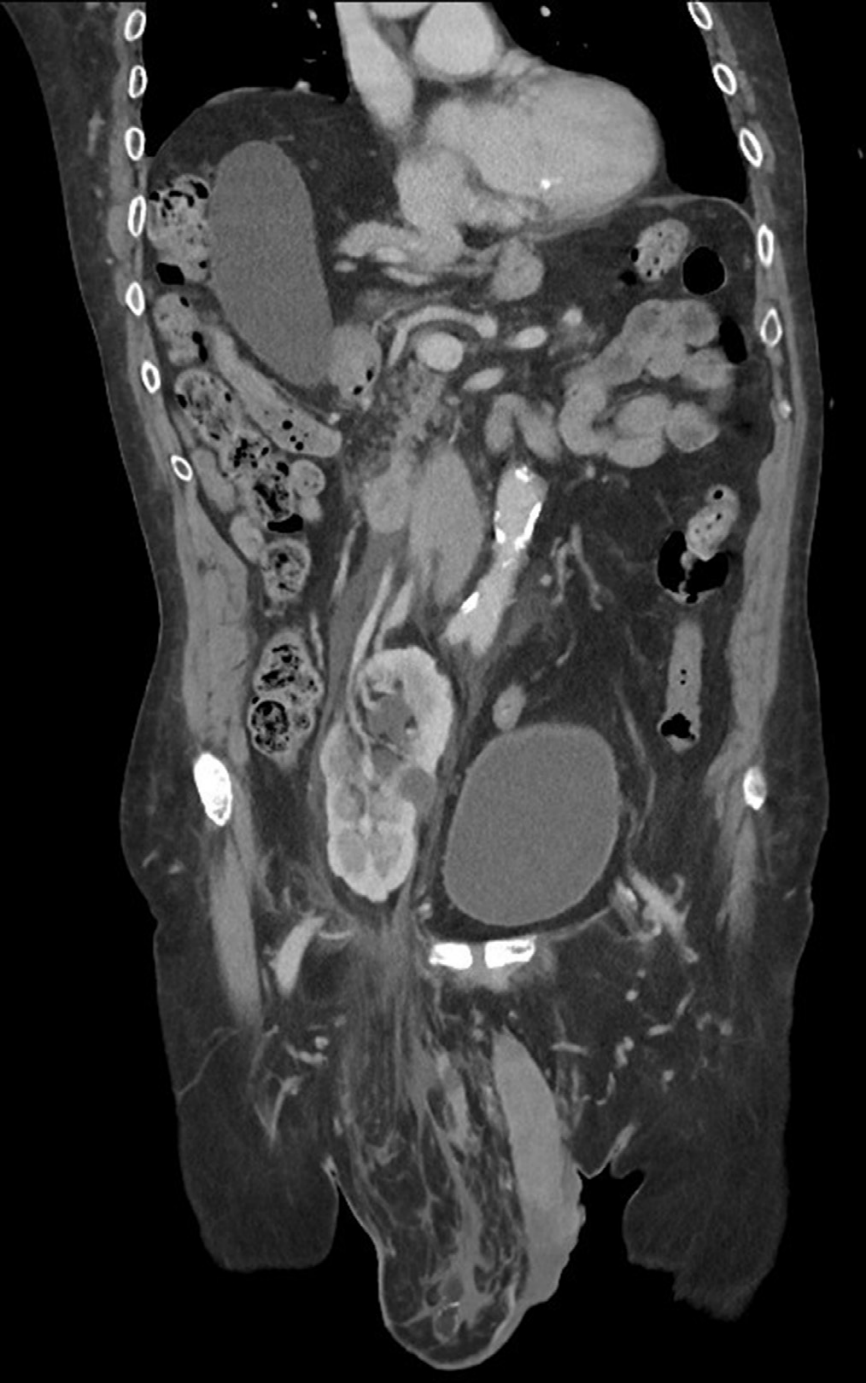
CT scan from 2019 depicting the large right inguinoscrotal hernia containing the tip of the lower pole of the right kidney with increased peri-nephric and hernial sac inflammatory changes as shown on the sagittal view.
